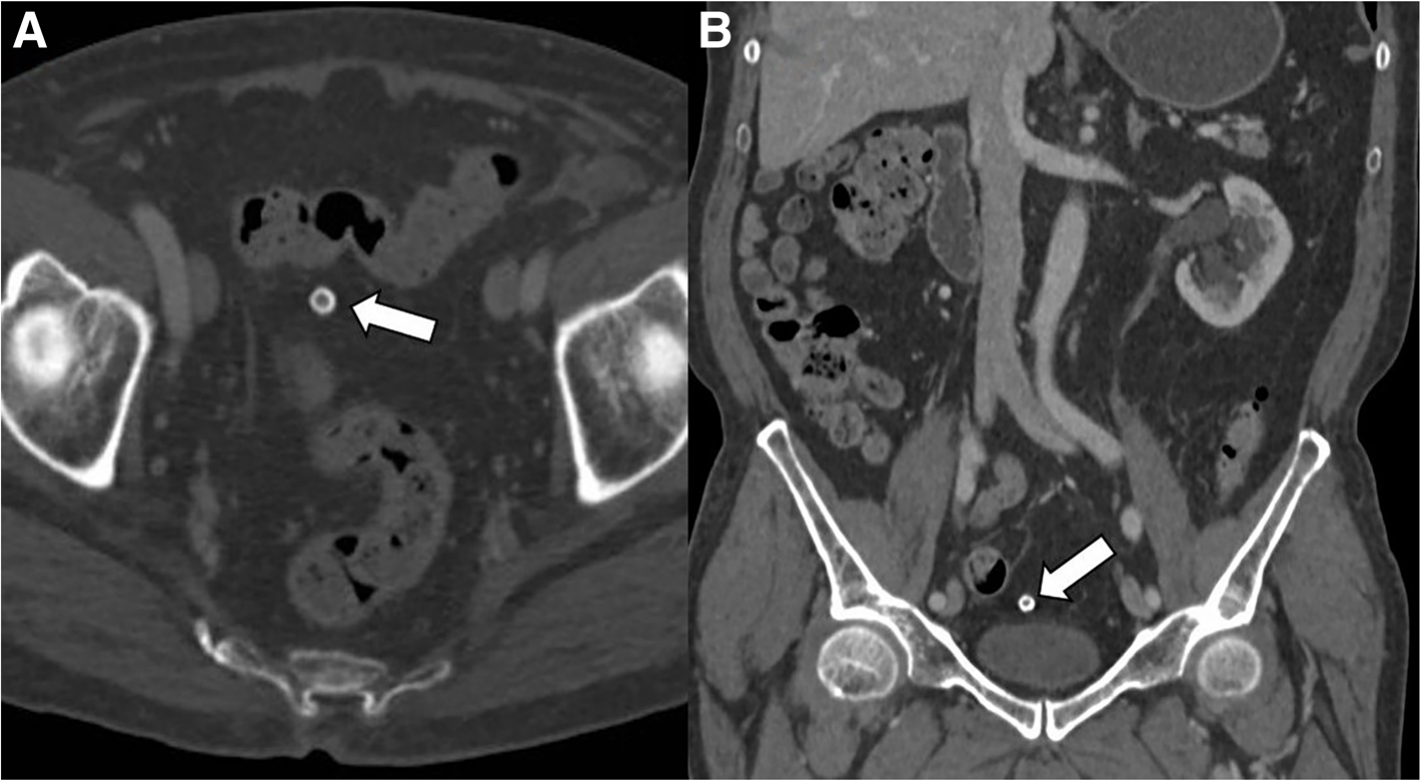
Chronic calcified, amputated epiploic appendage in an 81-year-old man. a Axial contrast-enhanced CT image (bone window) shows a calcified, ring-like lesion (arrow) medial to the sigmoid colon which could be mistaken for a mesenteric lymph node or drop gallstone. b Coronal reformatted CT image of the same patient depicts the peritoneal loose body in pelvic cavity, likely due to chronic infarcted epiploic appendage (arrow)
